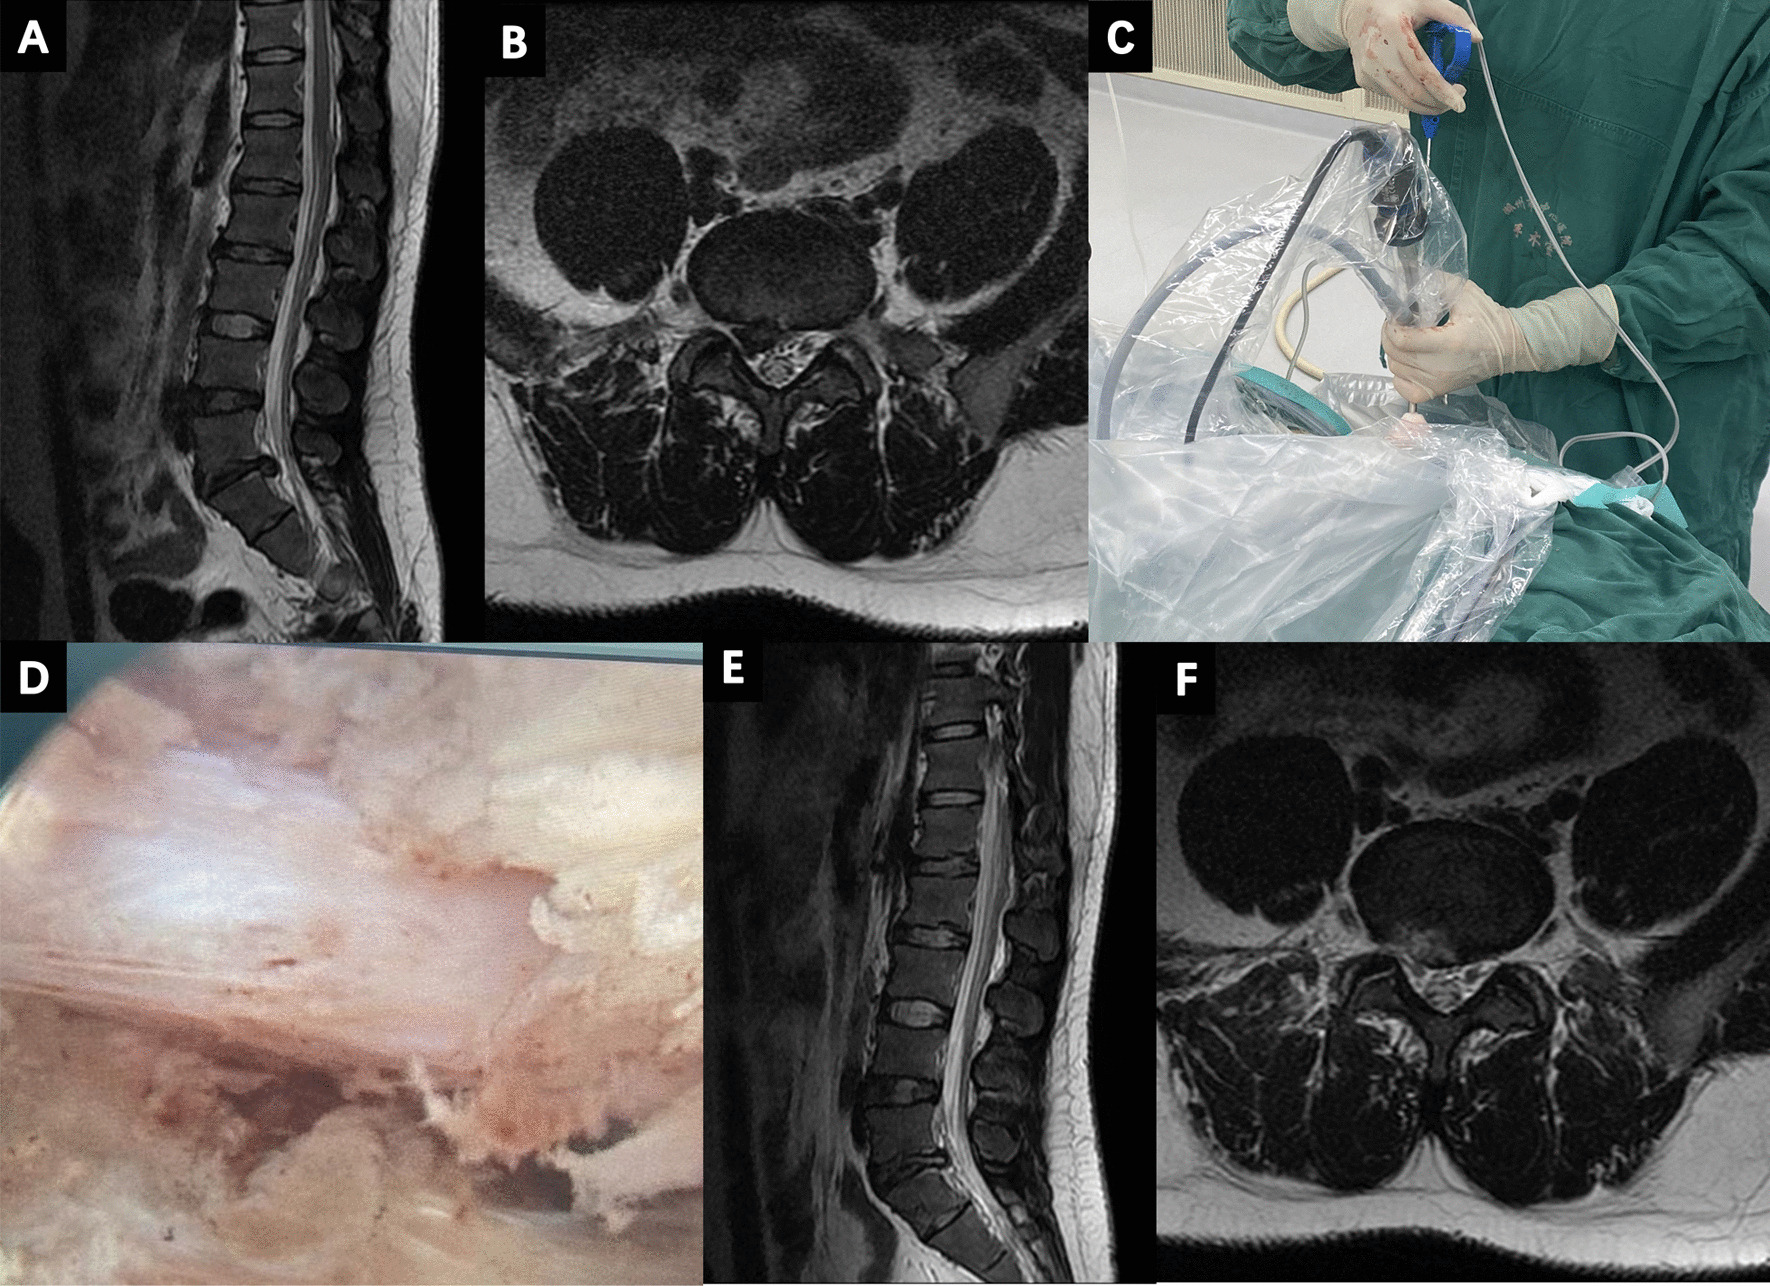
A, B Preoperative sagittal and axial magnetic resonance images in a 29-year-old male patient complaining of right radicular leg pain, showing L5-S1 disc herniation on the right side. C Image of the patient who underwent percutaneous endoscopic lumbar discectomy. D Endoscopic image showing the relaxation of S1 nerve root after decompression. E, F Postoperative sagittal and axial magnetic resonance images made one day after surgery
